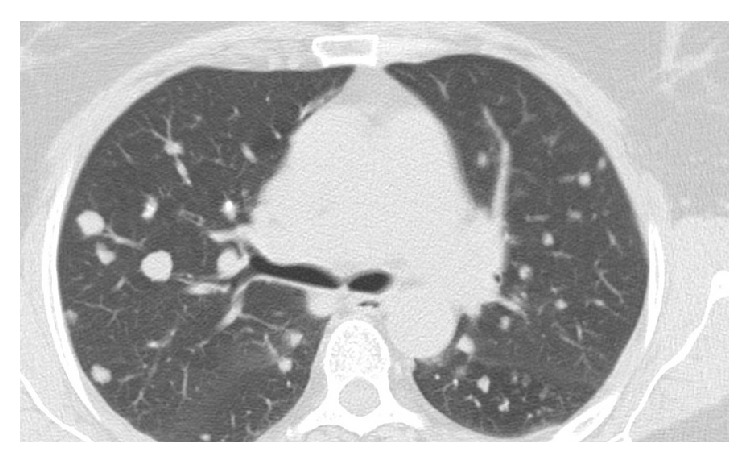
Bilateral multiple nodules with regular margins are detected in the CT of the patient.
